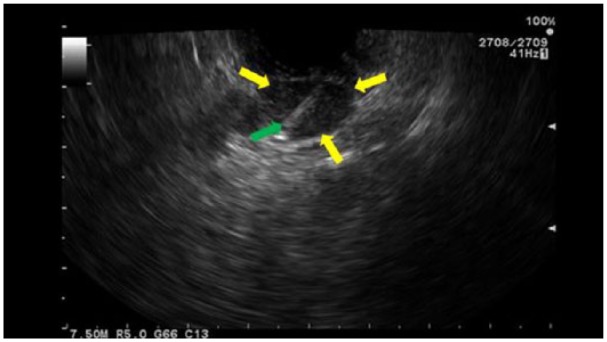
Endosonographic view of the enlarged celiac lymph node. Description: Fine needle aspiration of celiac lymph node. Green arrow is the needle, yellow arrows is the lymph node.
